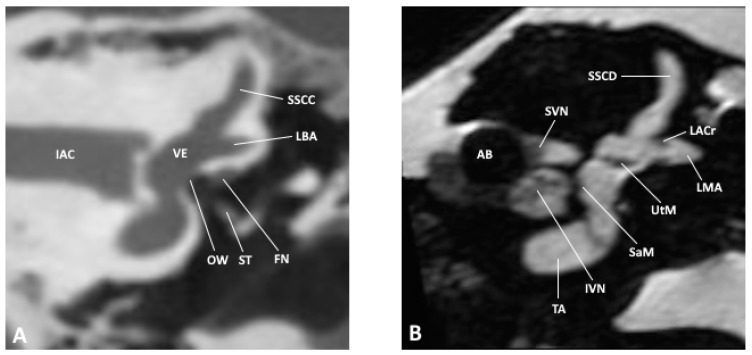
Coronal slice through the inner and middle ear at the level of the internal auditory meatus as observed by (A) CBCT and (B) MRI; AB, air bubble; FN, facial nerve; IAC, inner auditory canal; IVN, inferior vestibular nerve; LACr, lateral ampullary crest; LBA, lateral bony ampulla; LMA, lateral membranous ampulla; OW, oval window; SaM, saccular macula; SSCC, superior semicircular canal; SSCD, superior semicircular duct; ST, stapes; SVN, superior vestibular nerve; TA, truncation artifact; UtM, utricular macula; VE, vestibule.
